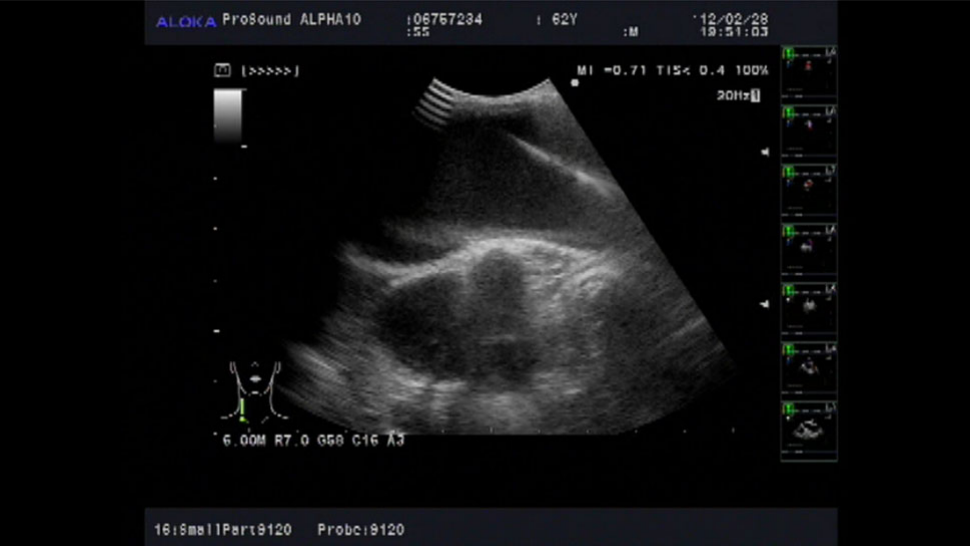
To ensure correct positioning of the tip of the arterial cannula in the true lumen by epiaortic ultrasound guidance
